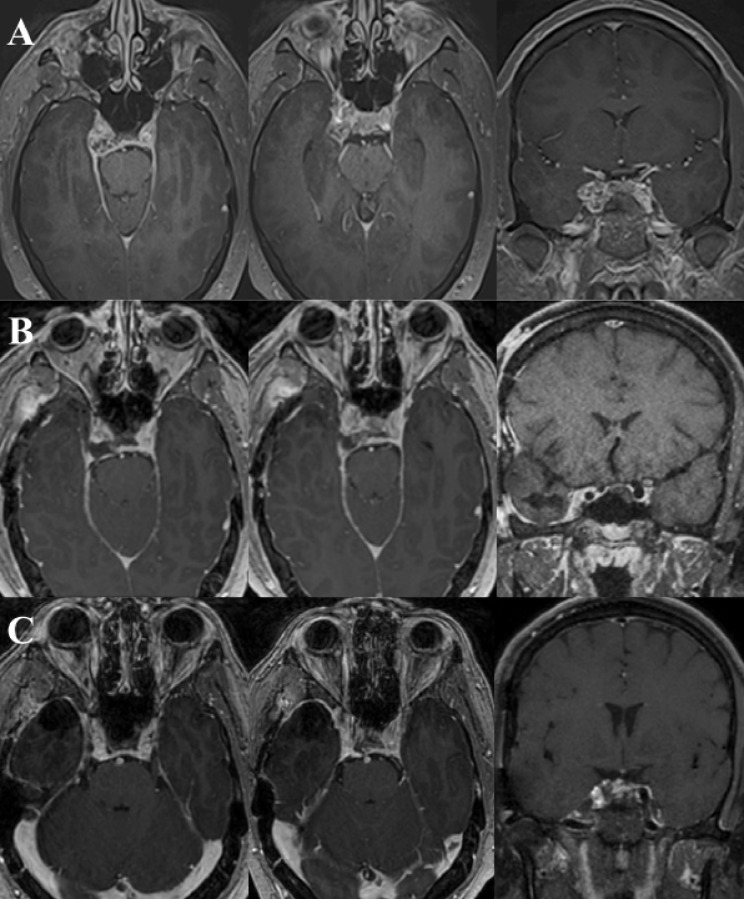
(A) Preoperative T1-weighted contrast-enhanced magnetic resonance image (MRI) of brain demonstrating axial and coronal views of a well-demarcated enhancing lesion within right cavernous sinus. (B) Postoperative axial and coronal views showing minimal residual enhancement along the right cavernous sinus. (C) Four-year follow-up MRI demonstrating stable appearance of the residual lesion with no evidence of progression.
